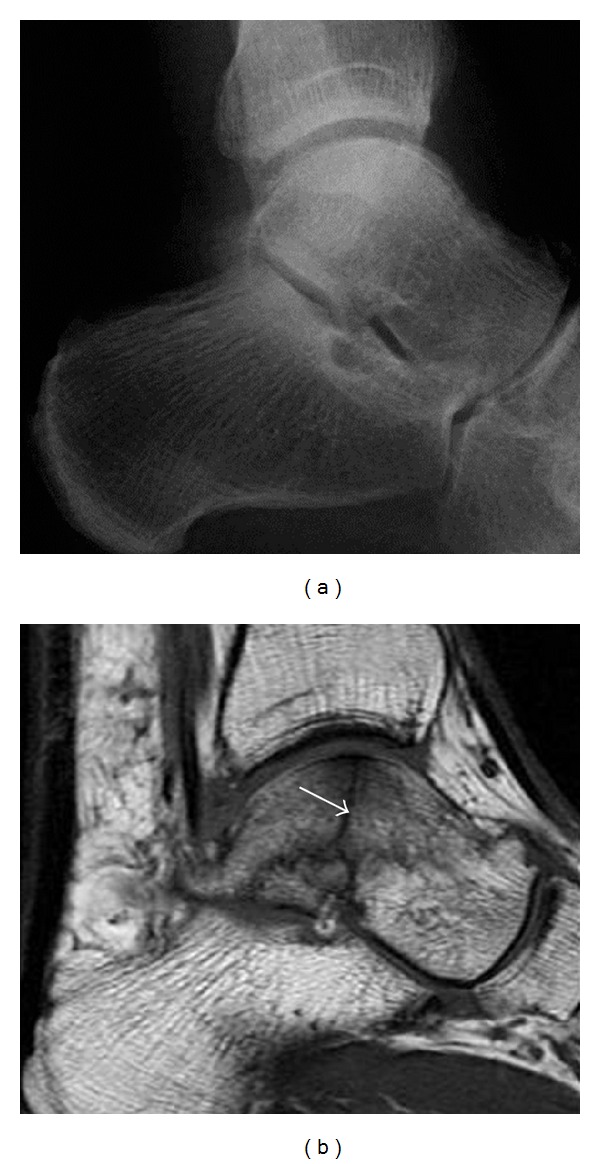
Fatigue fracture of the talus in a 25-year-old male basketball player with right hind foot and ankle pain, without history of trauma, and a normal initial radiograph (not shown). (a) One-month followup lateral radiograph shows normal appearance. (b) Sagittal T1-weighted MRI shows an irregular fracture line (arrow) within an ill-defined area of hypointensity corresponding to bone marrow edema.
